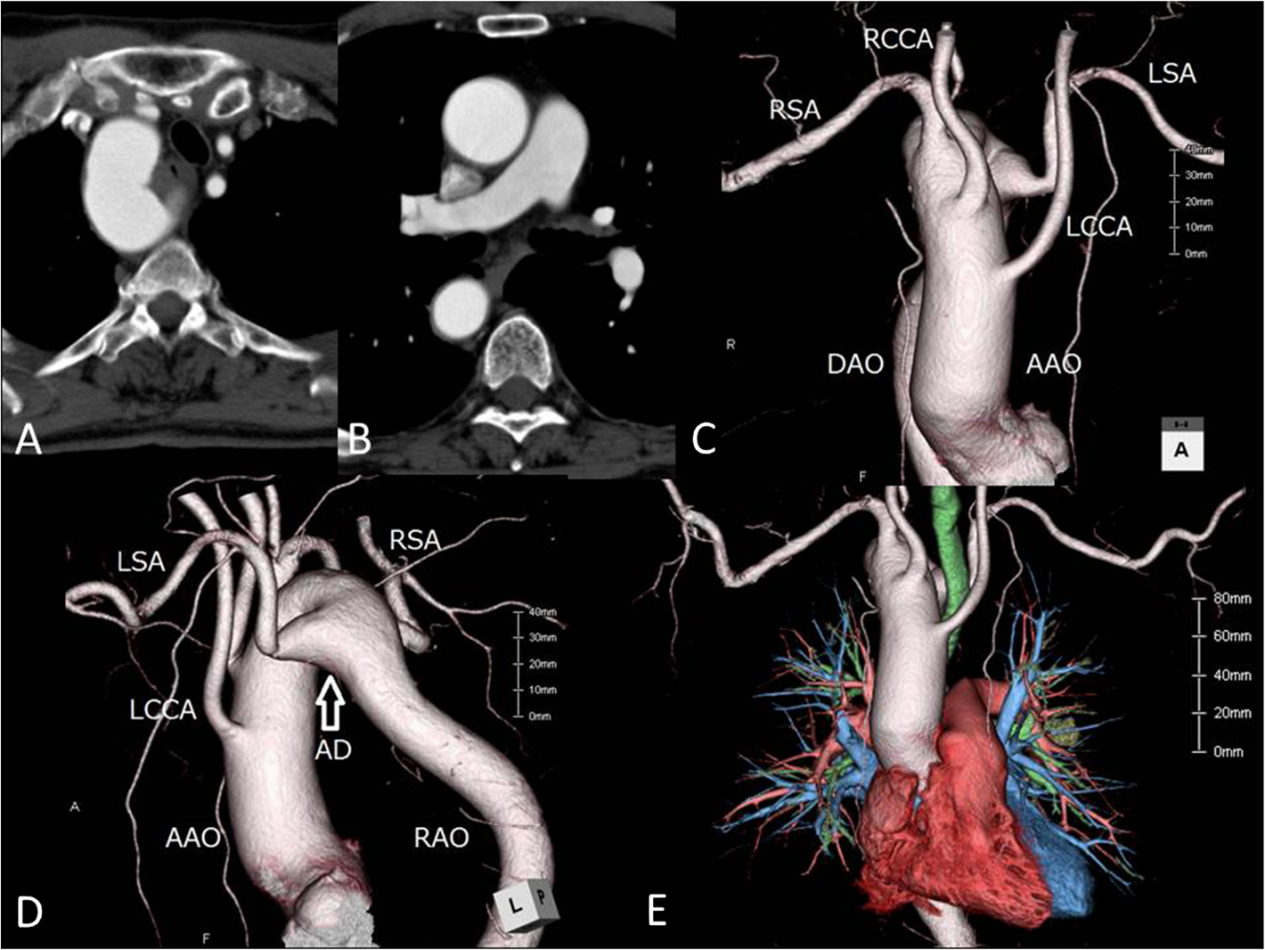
A chest computed tomography scan. A) The axial view shows the right aortic arch. B) The coronal view shows the right descending aorta in the right thoracic cavity. C) The anterior view of the three-dimensional computed tomography scan shows the right aortic arch with the aberrant left subclavian artery. D) The lateral view shows the so-called “Kommerell diverticulum” of the left subclavian artery. E) The branching of the pulmonary artery, vein, and the bronchi are normal. AAO, ascending aorta; AD, aortic diverticulum; DAO, descending aorta;. LCCA, left common carotid artery; LSA, left subclavian artery; RAO, right aorta; RCCA, right common carotid artery; RSA, right subclavian artery.
